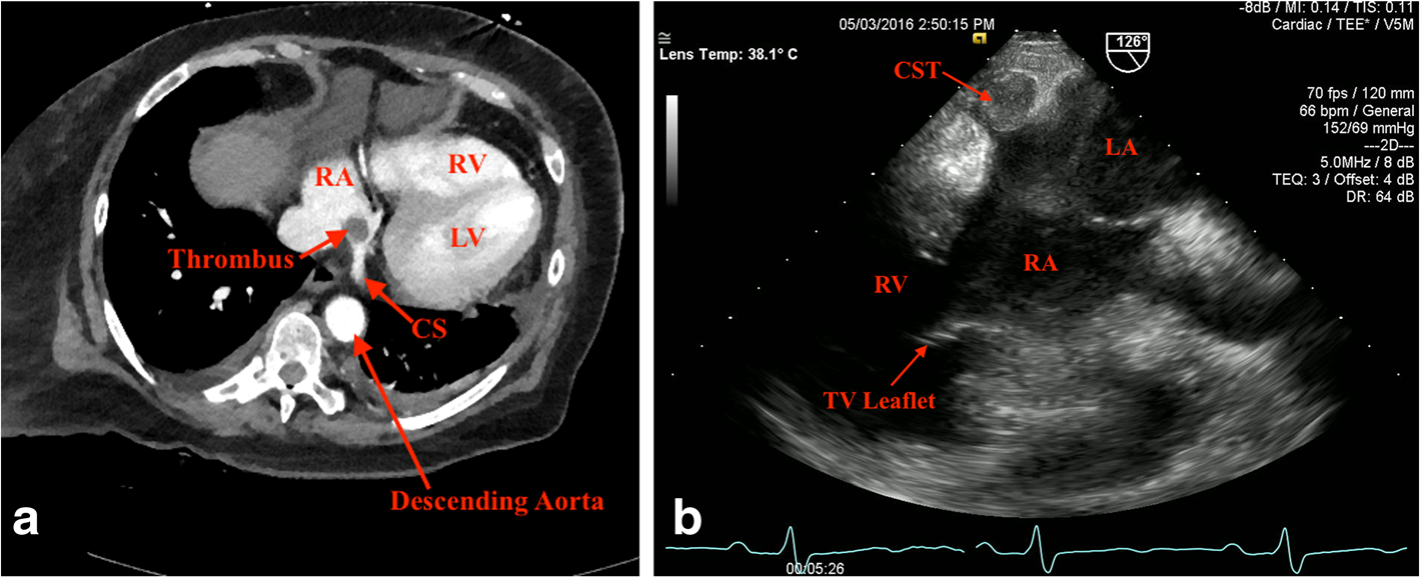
a Chest computed tomography with contrast at the level of coronary sinus showing an oval-shaped filling defect at the junction of the right atrium and coronary sinus suggestive of coronary sinus thrombus. b Transesophageal echocardiogram at the gastroesophageal junction positioned at 126° demonstrating a 1.5 × 1.5 cm echogenic globular mass at the ostium of the coronary sinus. *CS* coronary sinus, *CST* coronary sinus thrombus, *LA* left atrium, *LV* left ventricle, *RA* right atrium, *RV* right ventricle, *TV* tricuspid valve
